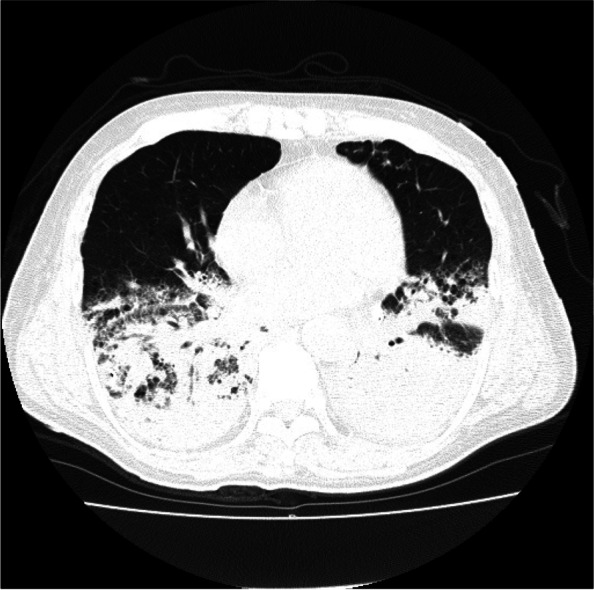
CT on the 5th POD revealed diffuse infiltration shadows in the bilateral lower lobe of the lung
